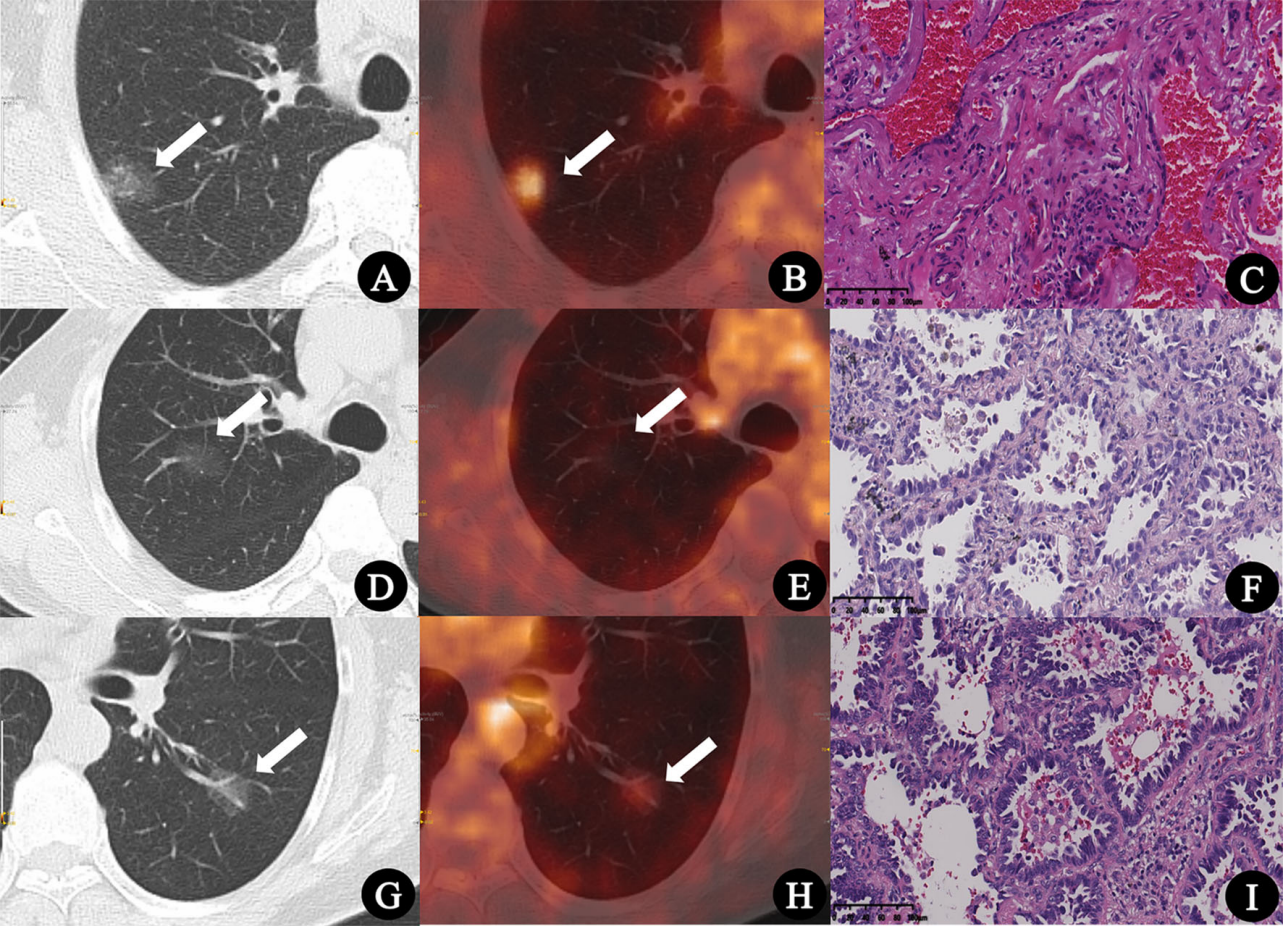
(A–C) Inflammatory pseudotumor in a 77-year-old man. (A) CT lung window image showed a pure ground-glass nodule with a diameter of 22.1 mm in the right upper lobe (arrow). (B) PET/CT fusion image showed that the SUV_max_ of the lesion was 3.2, and the SUV index was 1.28 (arrow). (C) Pathologic findings indicated fibrous tissue hyperplasia, hyperemia, and inflammatory cell infiltration (HE × 200). (D–F) Minimally invasive adenocarcinoma in a 74-year-old man. (D) CT lung window image showed a pure ground-glass nodule with a diameter of 20.9 mm in the right upper lobe (arrow). (E) PET/CT fusion image showed that the SUV_max_ of the lesion was 0.6, and the SUV index was 0.22 (arrow). (F) Pathologic findings indicated well-differentiated minimally invasive adenocarcinoma (HE × 200). (G–I) Invasive adenocarcinoma in a 55-year-old woman. (G) CT lung window image showed a pure ground-glass nodule with a diameter of 18.8 mm in the left lower lung lobe (arrow). (H) PET/CT fusion image showed that the SUV_max_ of the lesion was 1.4, and the SUV index was 0.52 (arrow). (I) Pathologic findings indicated moderately differentiated invasive adenocarcinoma (HE × 200).
